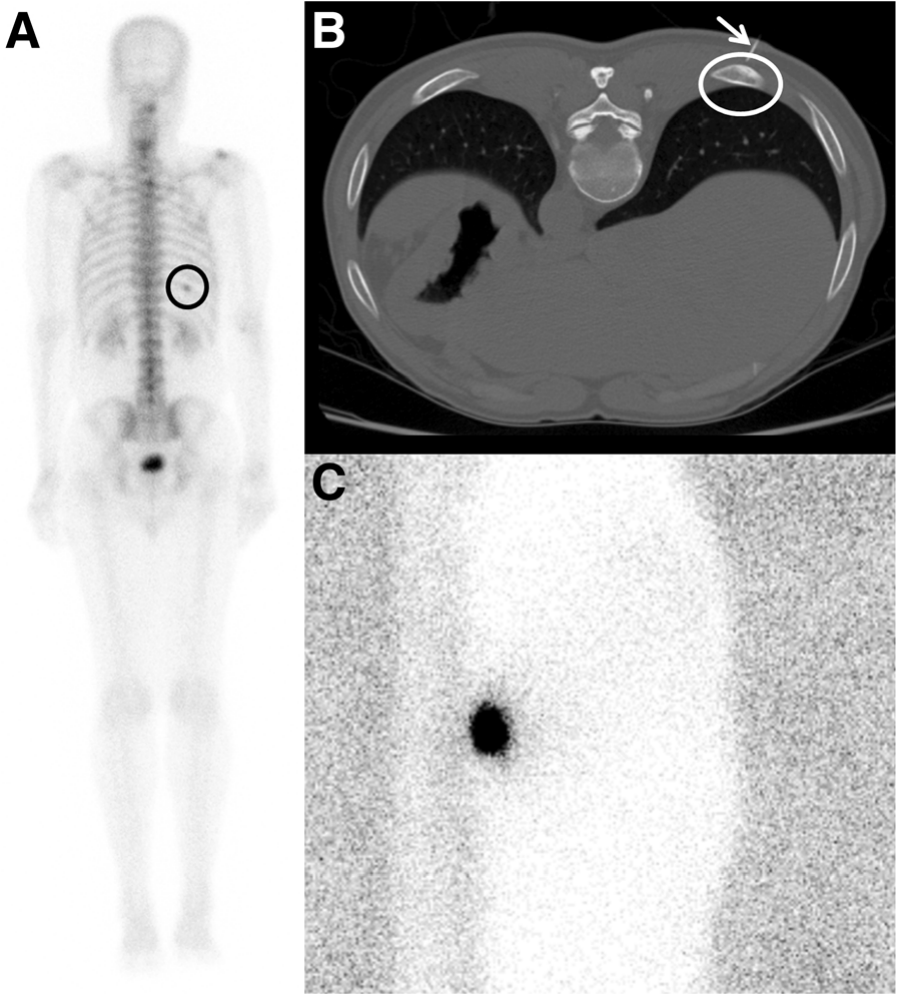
A 60-year-old male, with history of treated Gleason 7 prostate carcinoma presenting with a rising prostate-specific antigen (PSA). The only active site of disease was a posterior right 10th rib lesion (black circle) identified on a Tc-99m methyl diphosphonate bone scan (a). This lesion underwent CT-guided radiolocalization (b) where a 9-mm sclerotic rib lesion (white circle) is being injected with Tc-99m MAA using a percutaneous needle (white arrow). A right lateral scintigram (c) depicts focal radiotracer in the region of targeted rib lesion. This portion of the right 10th rib was surgically excised and pathology confirmed metastatic prostatic adenocarcinoma. Patient’s serum PSA subsequently dropped from 29.3 to 3.7 ng/mL
